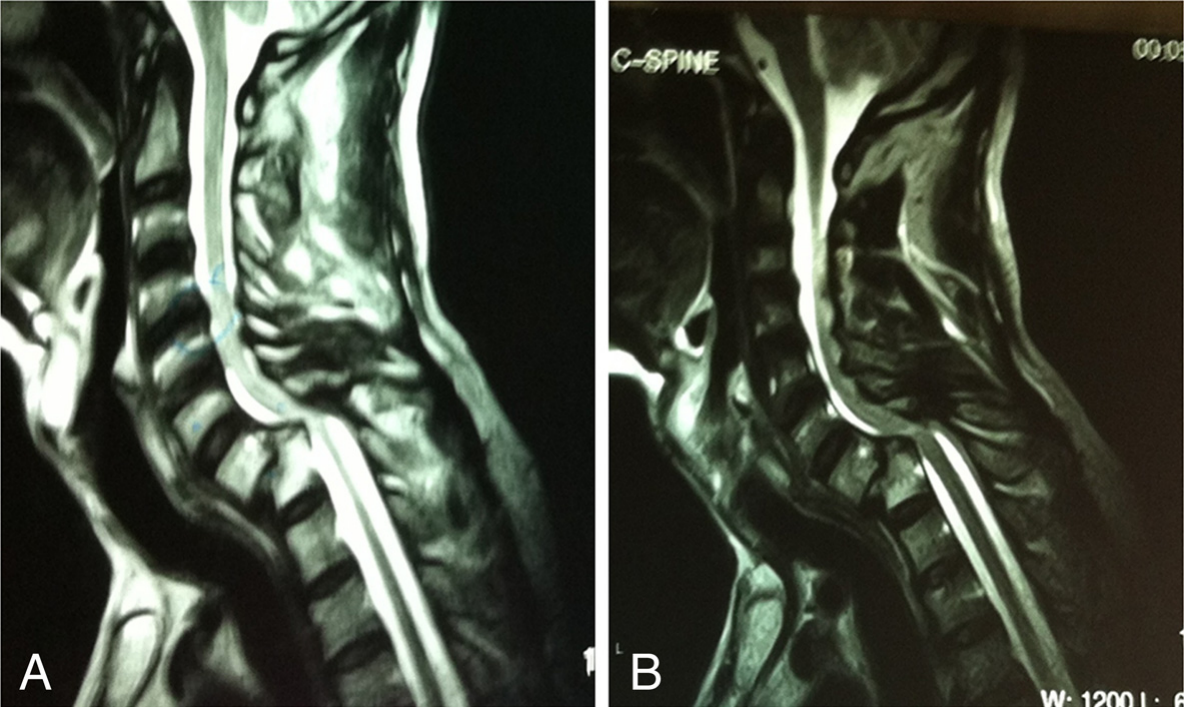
Computed tomography cervical spine sagittal images. Computed tomography images with (A) and without (B) contrast reveal severe cord compression at the level of C7.
